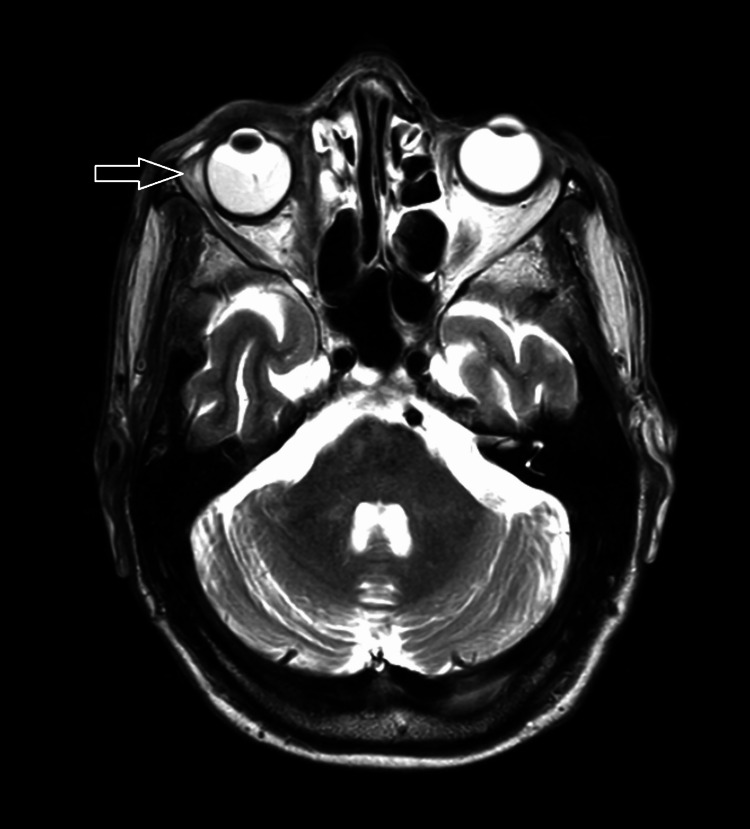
MRI taken 3 months after radiotherapy (T2-weighted image from the axial plane showing a decrease in the size of the right orbital process with intermediate signal).
